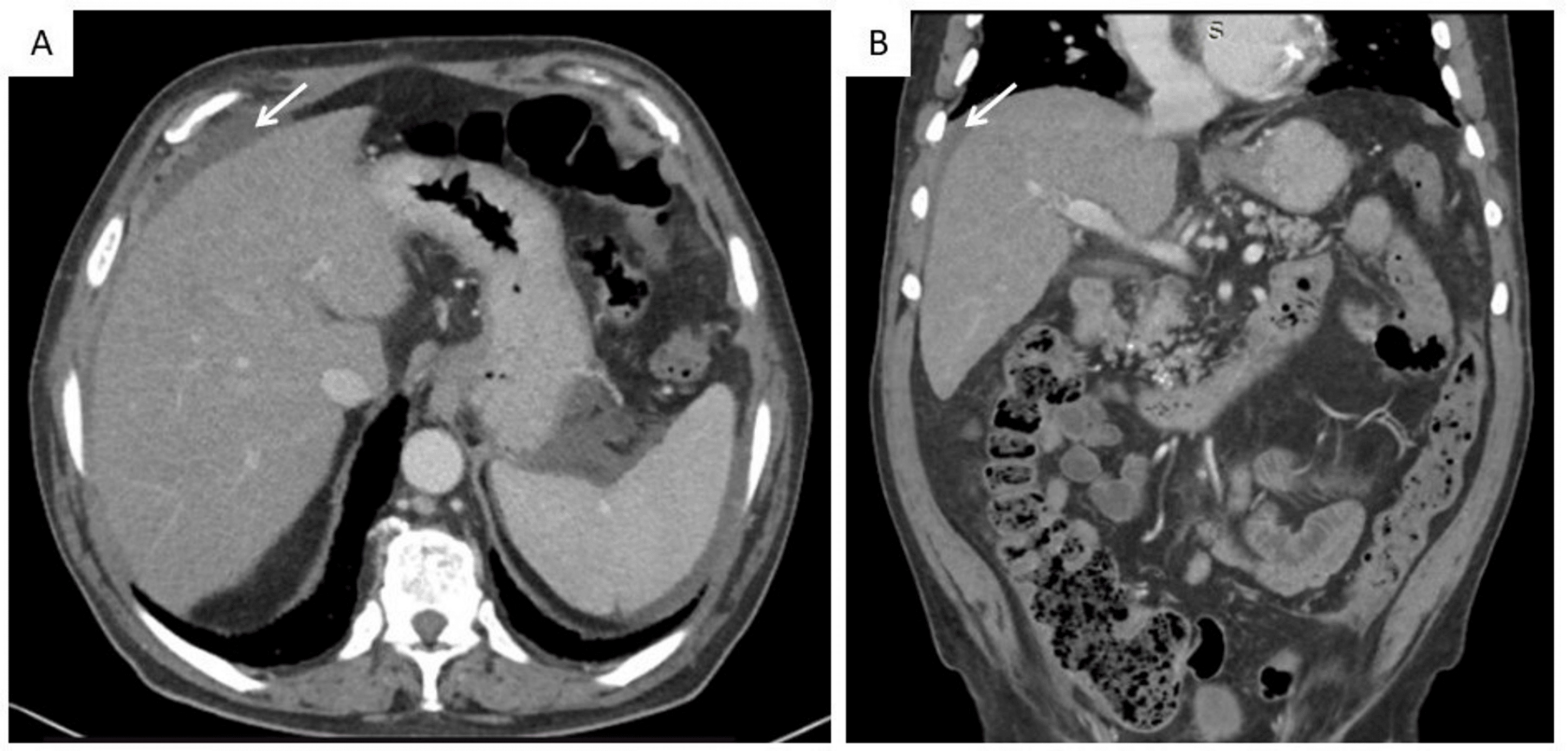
Thoraco-abdominopelvic computed tomography without signs of chronic liver disease but with small volume perihepatic ascites (white arrows) A) Axial plane; B) Coronal plane
